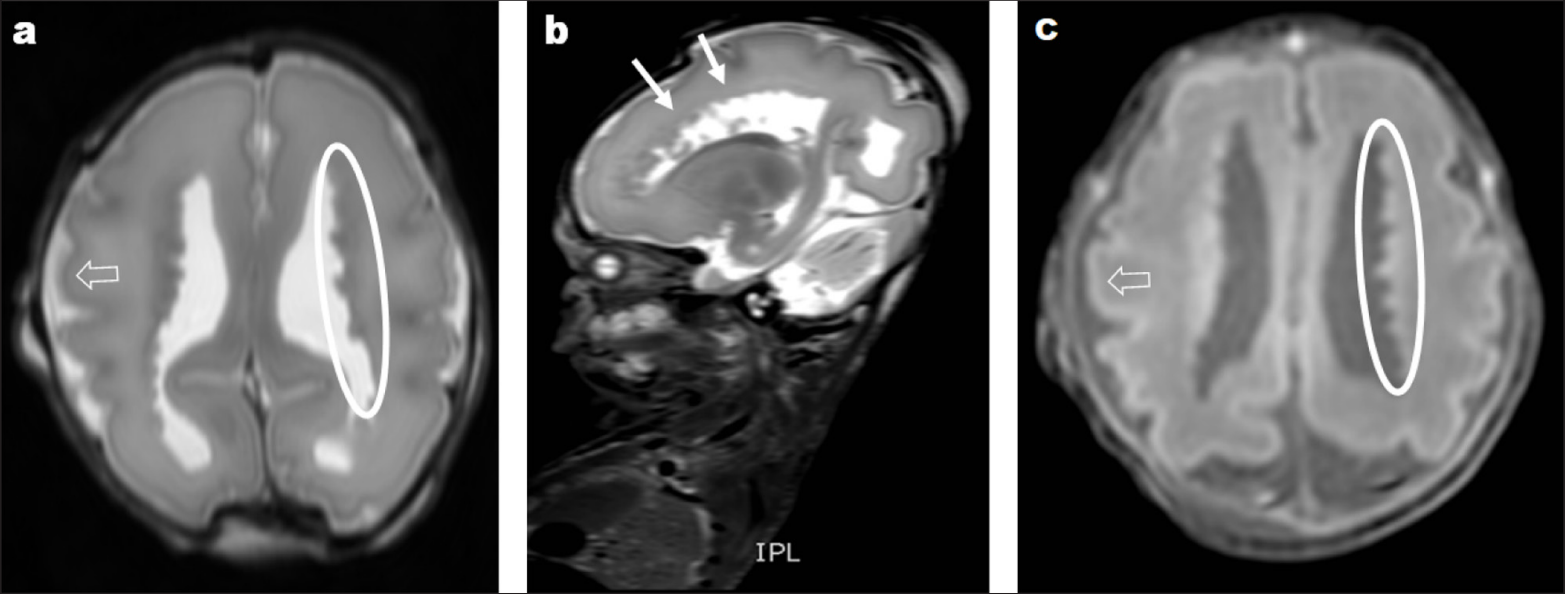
Post-mortem axial and sagittal T2w (a, b) and axial T1w (c) MR images of the brain at a fetal age of 27 weeks. The ventricles are lined with multiple nodules (closed arrows and ovals) following the signal intensities of the grey matter (open arrows).
